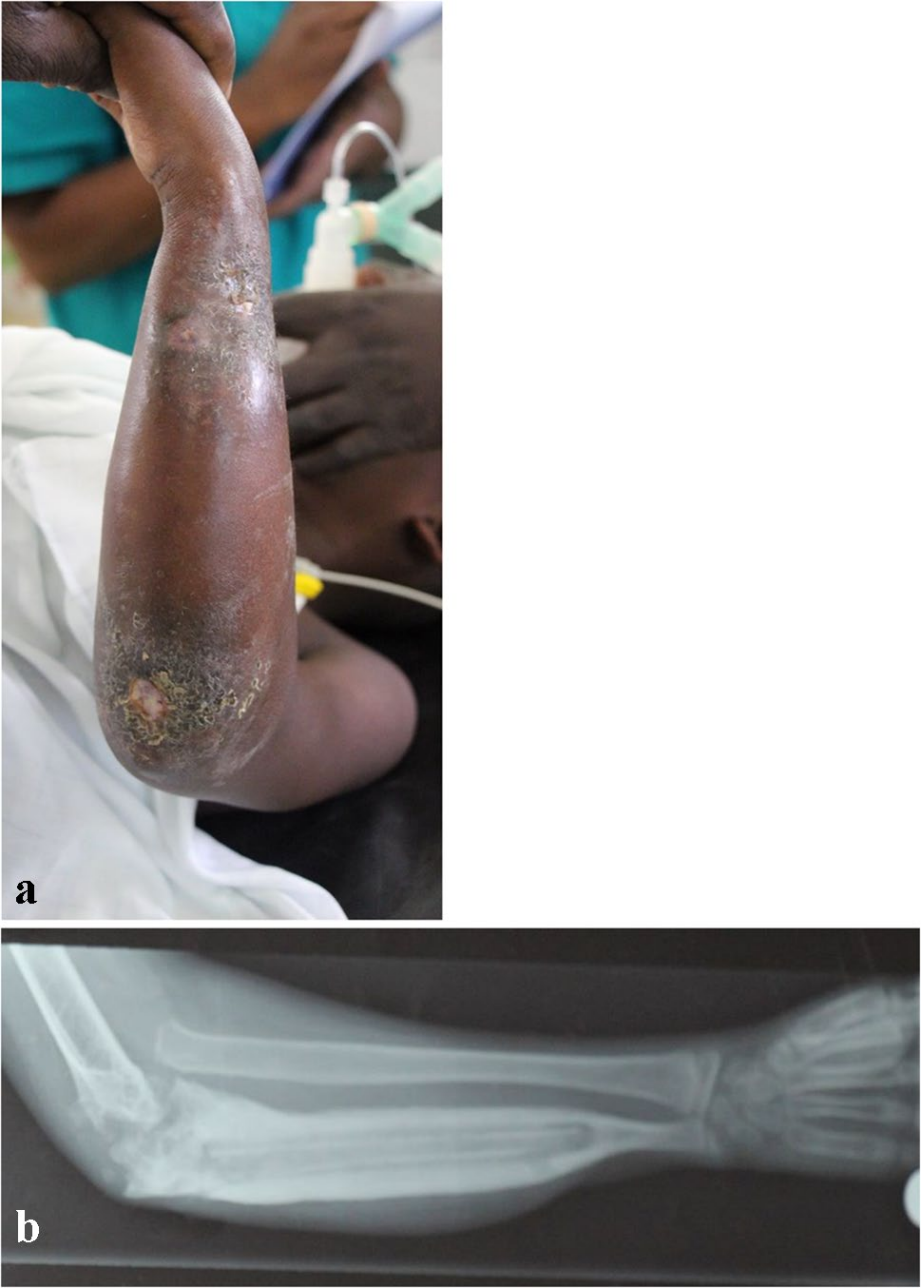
a clinical photo of a child with two areas of draining sinus due to underlying chronic osteomyelitis. b AP xray of the forearm demonstrating a large sequestrum in the ulna with surrounding involucrum. The radiocapitellar joint is dislocated and the ulnohumeral joint shows degenerative changes, consistent with an untreated septic arthritis
